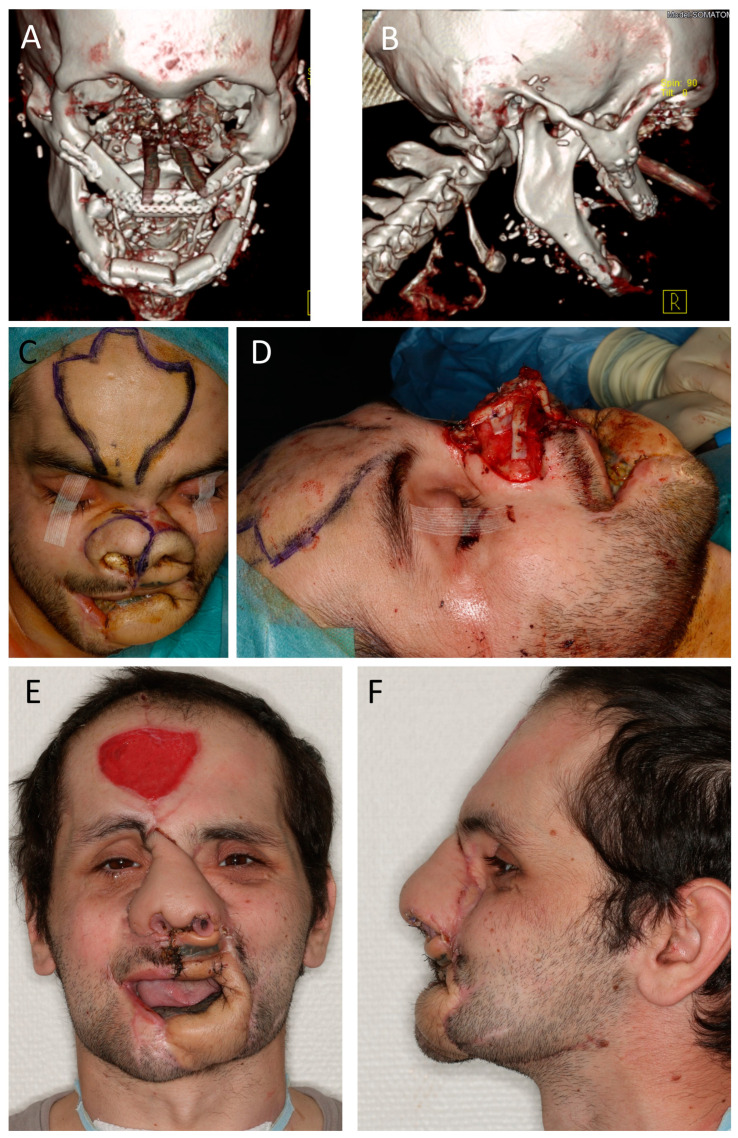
(Case 12, continued) Mid and lower face reconstruction. (A,B) CT scan showing bony fibula reconstruction of maxilla and mandible after steps 1 and 2. (C) Surgery three. Reconstruction of the skeletal base of the nose. Marking of the nasal hinge over flaps and the forehead flap. (D) Nasal framework reconstruction with iliac crest graft for osseous radix and rib graft for nose projection (columella, dorsum, and upper and lower lateral cartilage). (E,F) Situation 4 weeks after fourth surgery, showing projection of the mid face and good mouth opening. A wound-healing disturbance at the mid-upper lip was closed secondarily. The patient was able to eat, and the tracheostoma was removed on that day. After these steps, further refinements of the patient were denied by the government, and the patient was sent back to his home country.
